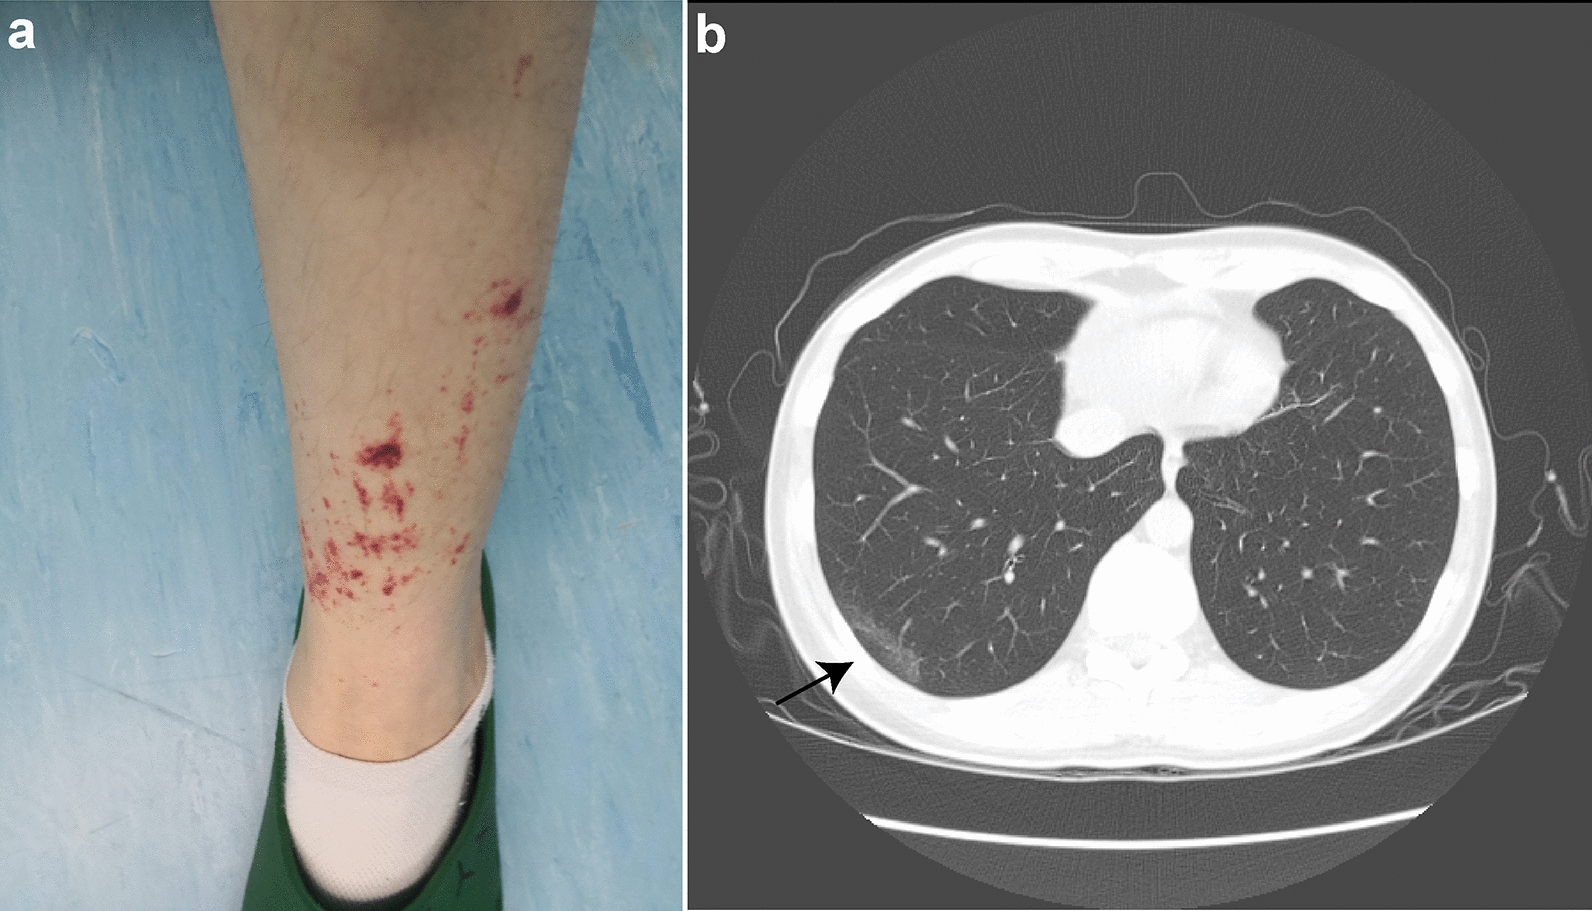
A Skin lesion presenting as purpura of the left lower limb. B Chest CT scan showed a subpleural patchy shadow in the posterior basal segment of the right lower lobe (black arrow)
